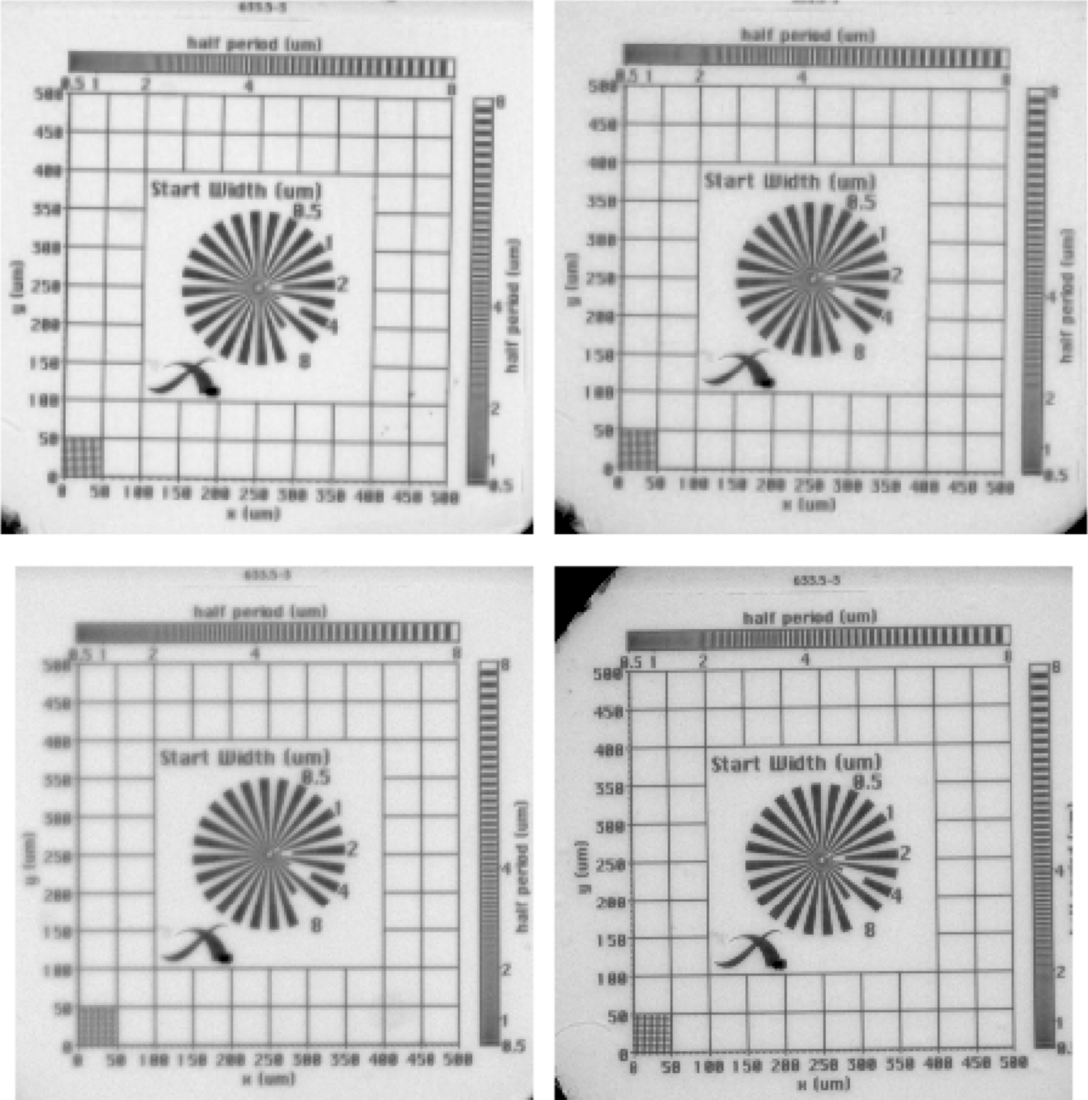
Characterization of the X-ray detector system utilizing the X-ray test pattern (Xradia Inc., model X500-200-30) and 26 keV single harmonic illumination (*t* stands for scintillator thickness). Top left: M Plan Apo with LYSO:Ce (*t* = 250 µm). Top right: M Plan Apo with LuAG:Ce (*t* = 250 µm). Bottom left: NUV M Plan Apo with LYSO:Ce (*t* = 250 µm). Bottom right: NUV M Plan Apo with LuAG:Ce (*t* = 250 µm).
